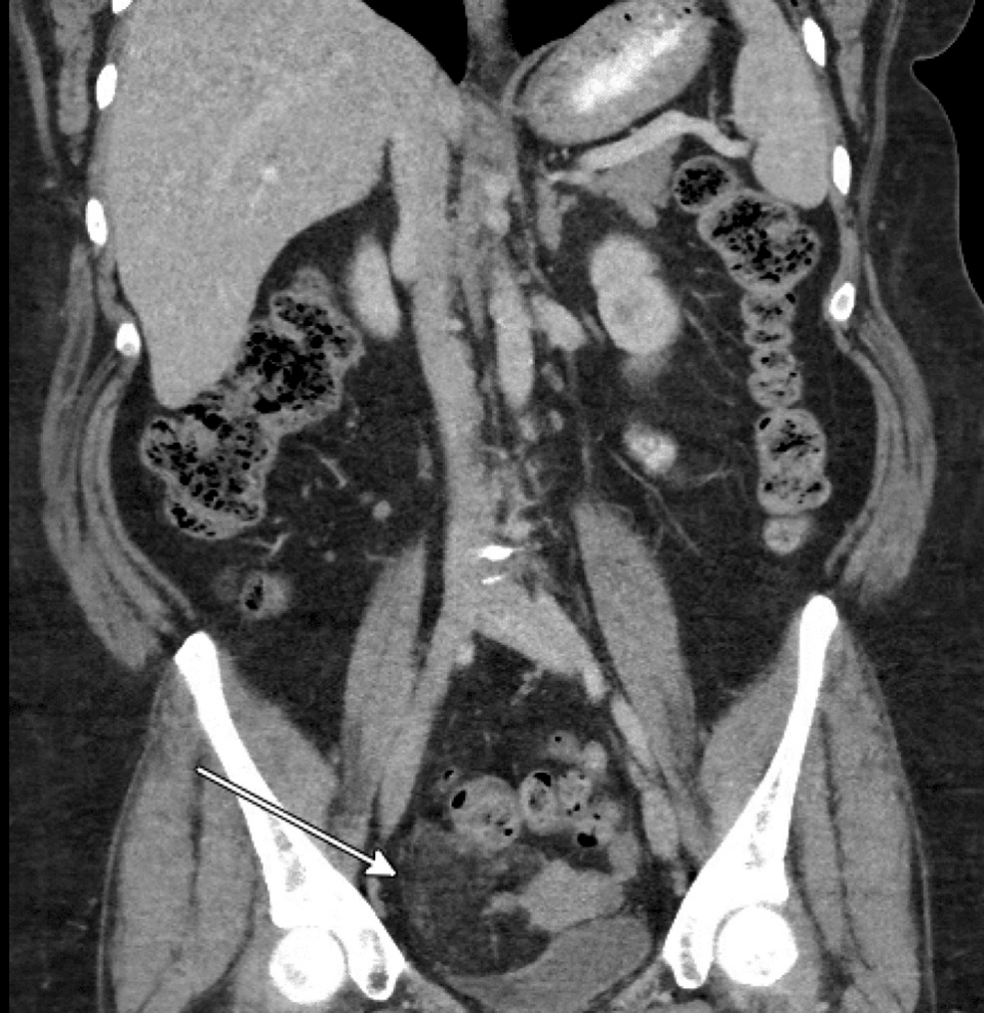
Radiographic recurrence of mass with an arrow depicting the area of concern
